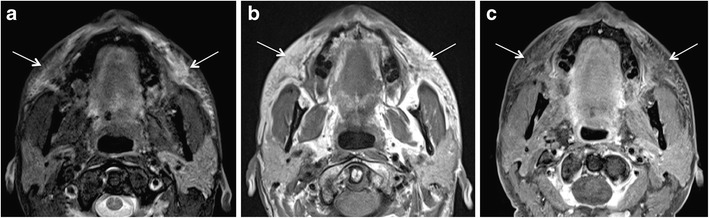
A 49-year-old woman developed bilateral painful facial swellings and redness of skin 4 months after bilateral facial injection of PAAG fillers. On MRI, ill-defined streaky areas of signal abnormalities, hyperintense on axial STIR (*arrows* in a) and iso- to hypointense on axial T1 W images (*arrows* in b), were seen in bilateral superior jowl and medial superficial cheek fat compartments with extension to bilateral medial deep cheek fat and buccal fat pads. These areas display reticulated enhancement on post contrast fat saturated T1 W images (*arrows* in c) suggesting cellulitis/infection. Haematological investigations showed raised inflammatory markers. Patient responded to treatment with antibiotics
